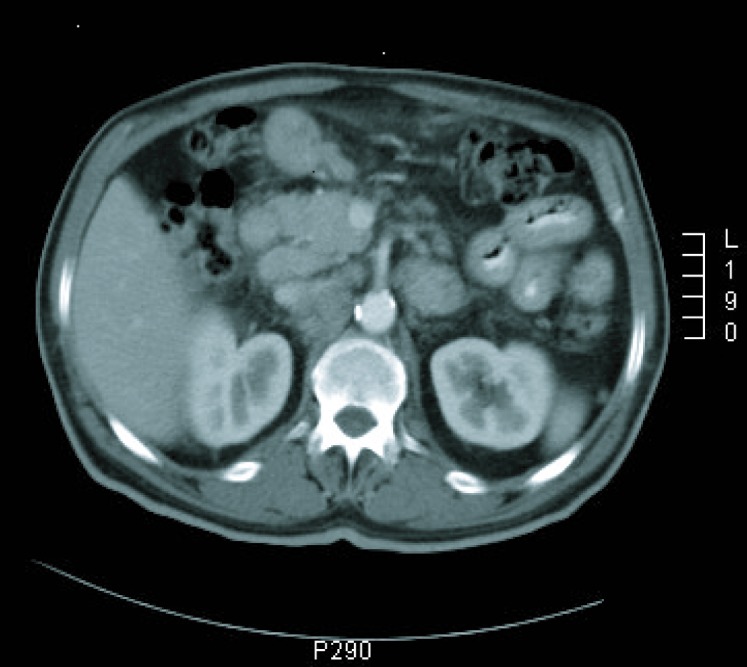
CT imaging at diagnosis.
